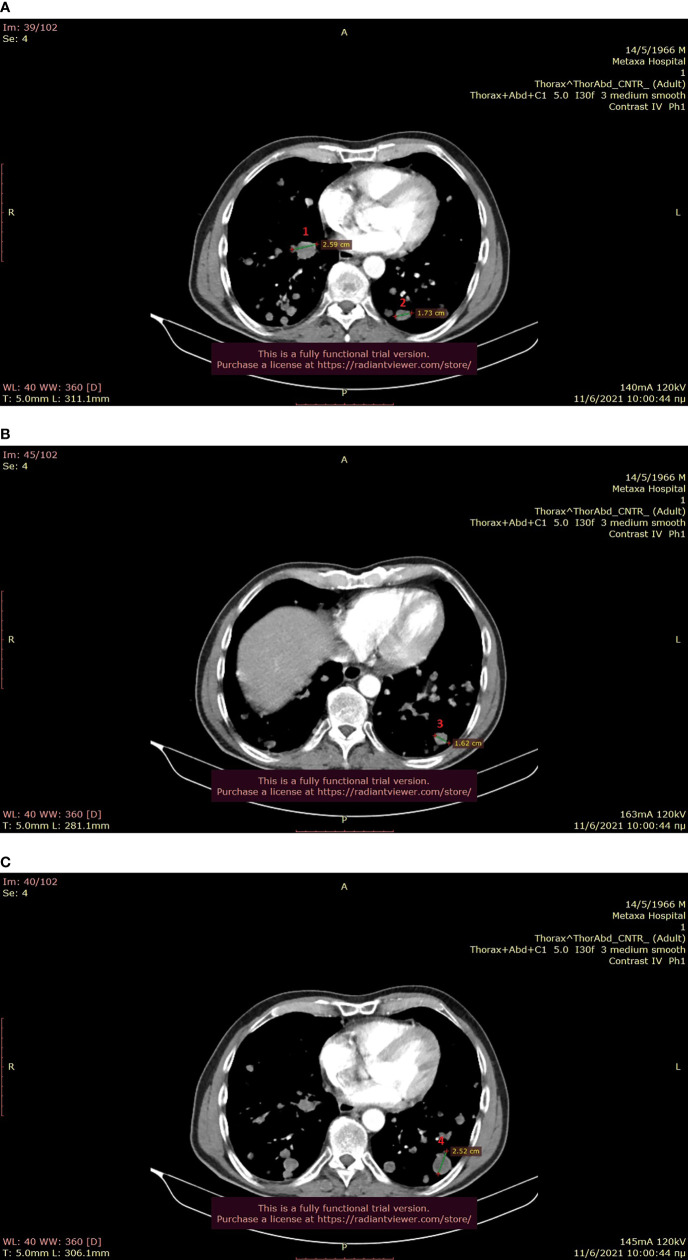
(A–C): Baseline thoracic CT showing multiple metastases bilaterally (June 2021).
